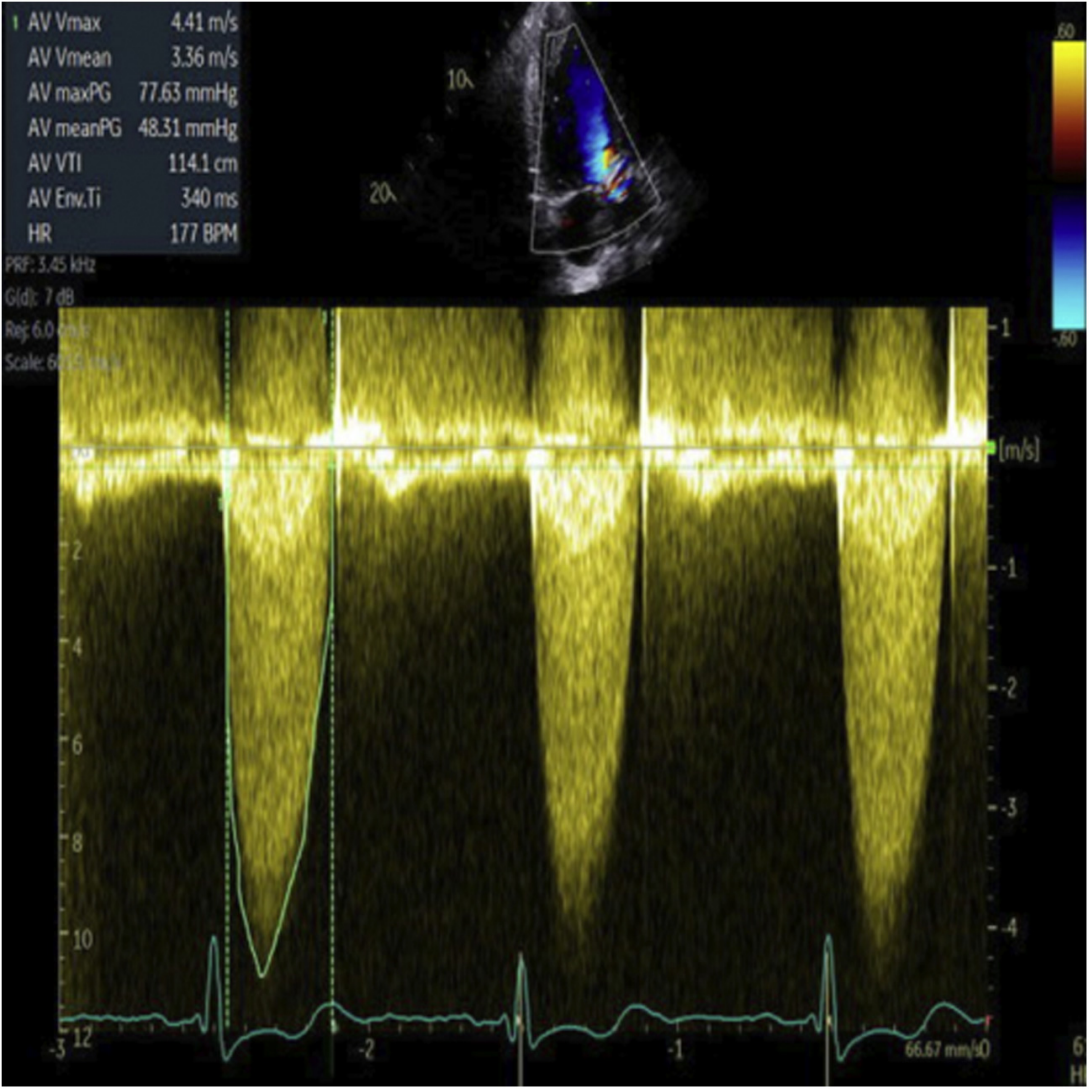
Severe aortic stenosis on transthoracic echocardiography: Aortic bioprosthetic stenosis with mean pressure gradient of 48 mm Hg.
